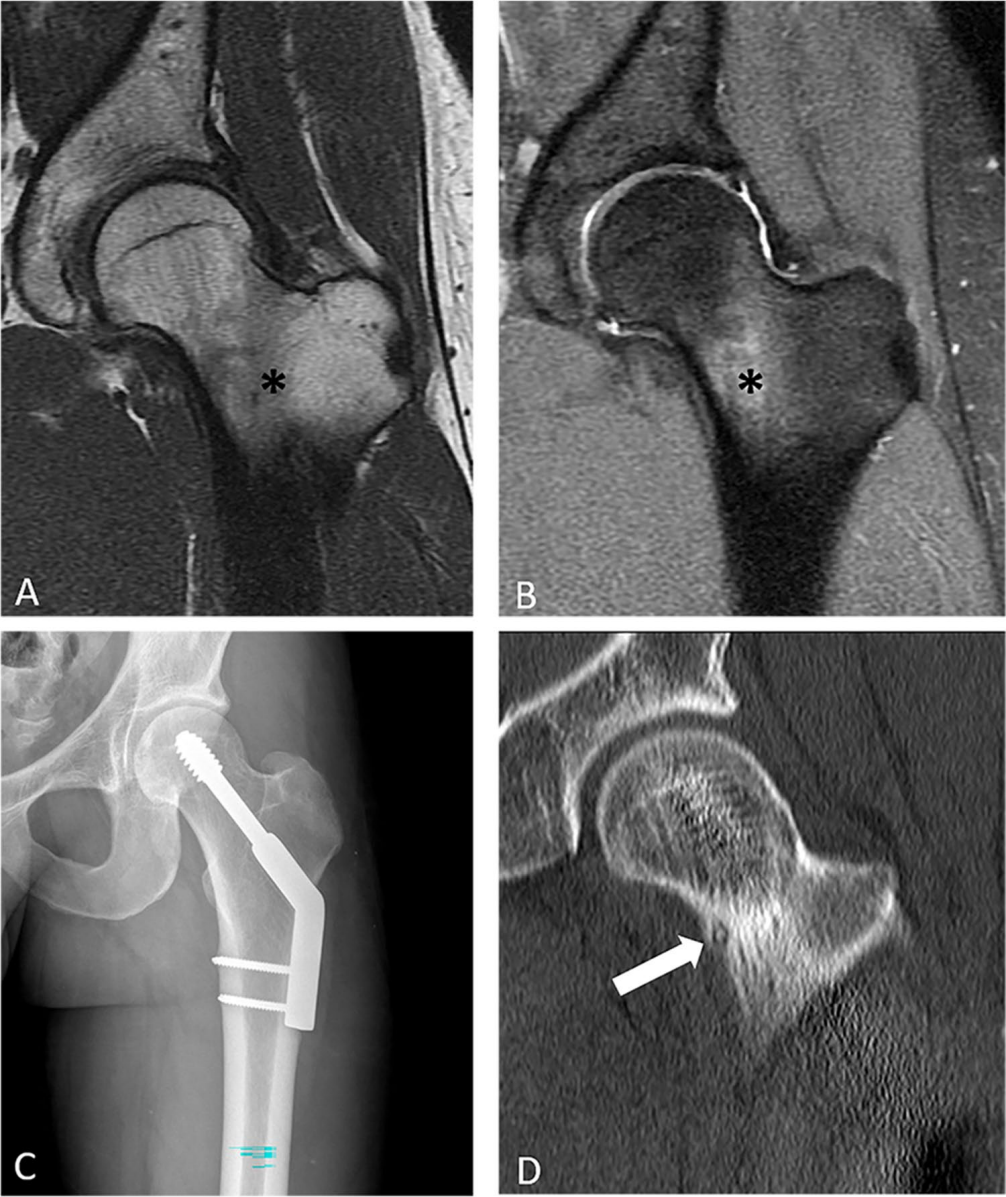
Coronal T1 (A) and STIR (B) MR images of the left hip of a 26-year-old male show bone marrow edema in the femoral neck (asterisk). Patient was diagnosed with a stress fracture and treated with dynamic hip screw fixation (C). Symptoms were unchanged postoperatively. Coronal CT obtained to evaluate for a source of pain and hardware complications (D) shows a tiny intracortical OO in the femoral neck anteriorly (arrow) that was successfully treated with RF ablation
